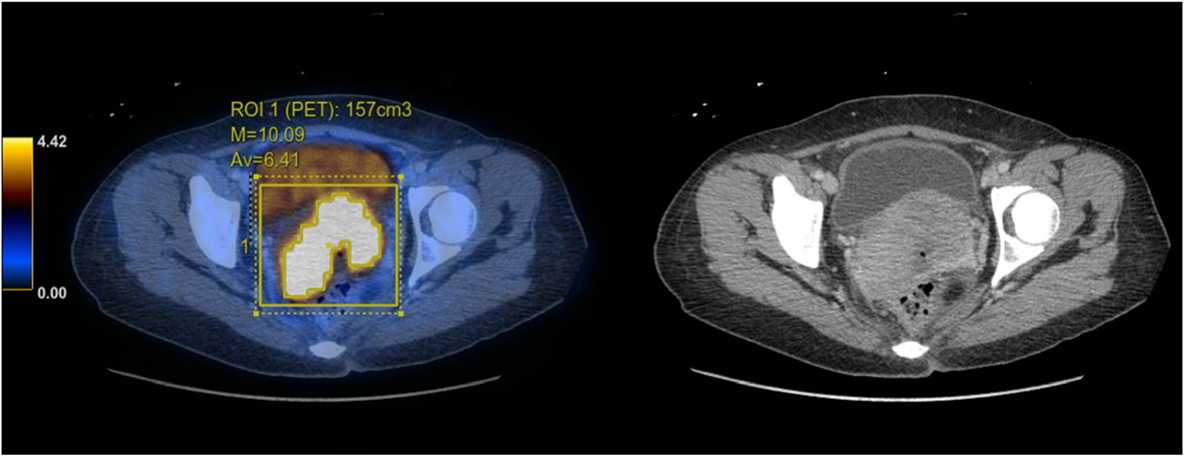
MTV was calculated by the thresholding method on FDG PET/CT. Focal activity in the uterine cervix was identified. A VOI was inserted manually, carefully avoiding the urinary bladder. SUVmax was quantified by the software automatically. The tumour was outlined as the region encompassed by a given fixed percent intensity level relative to the maximum activity in the tumour. 20% to 80% thresholds of the SUVmax (MTV_20_ to MTV_80_) at intervals of 5% were used in this study. MTV: metabolic tumour volume; VOI: volume of interest; SUVmax: maximum standardized uptake value
